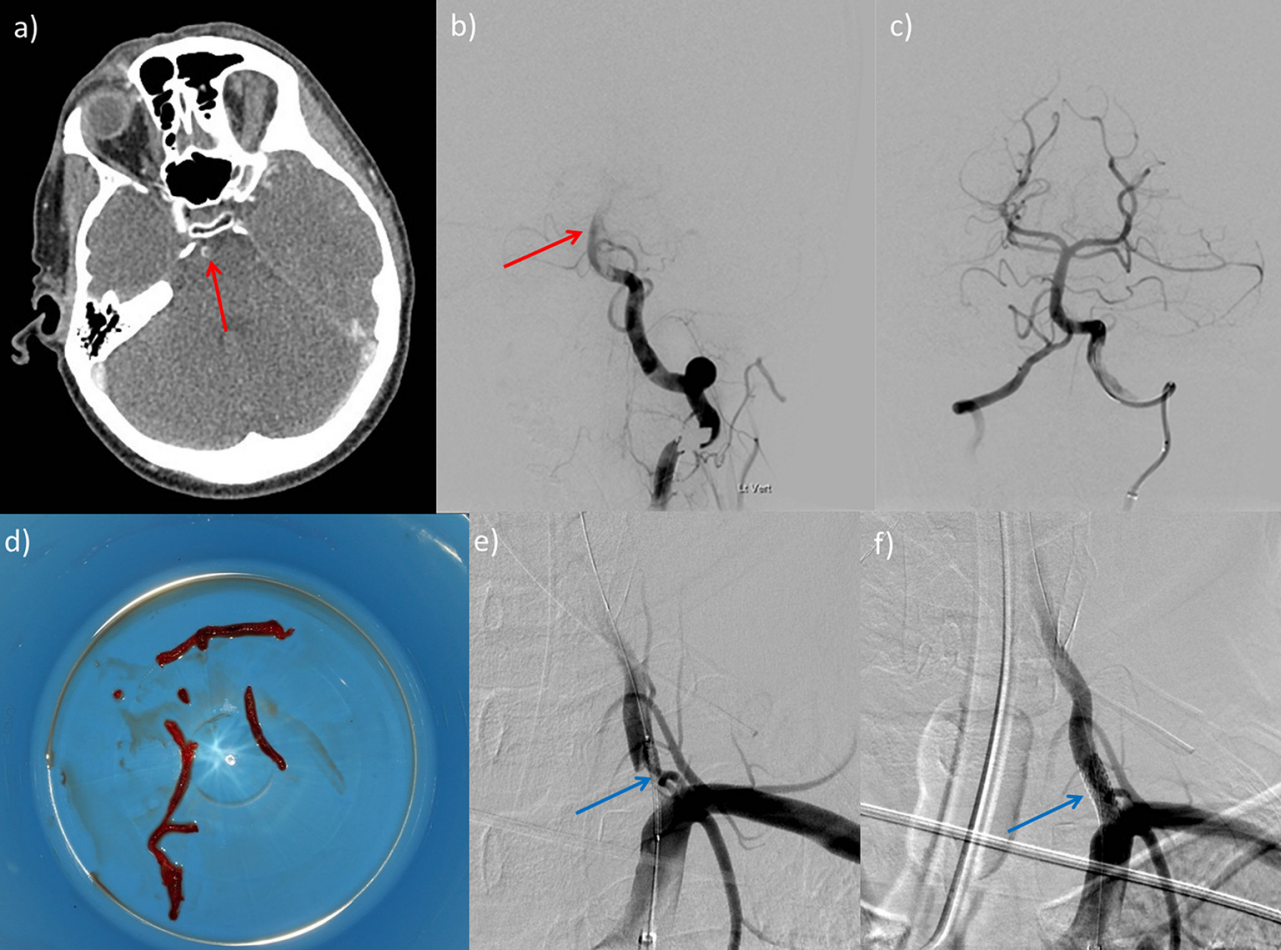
Plain CT scan of head (a) and prethrombectomy (b) and post-thrombectomy (c, e, f) digital subtraction angiograms in a 58-year-old man with a short history of visual symptoms and vertigo followed by a rapid drop in conscious level. Plain CT scan of head (a) shows thrombus in the basilar artery (red arrow) with complex plaque at the vertebral artery origin, confirmed on digital subtraction angiography (b). Following successful thrombectomy (c), with removal of a large cast of thrombus (d) by aspiration, a stent was deployed across the unstable stenotic plaque at the vertebral artery origin (blue arrows, e and f). Basilar thrombi can often be removed in bulk like this, possibly because of their physical composition.
